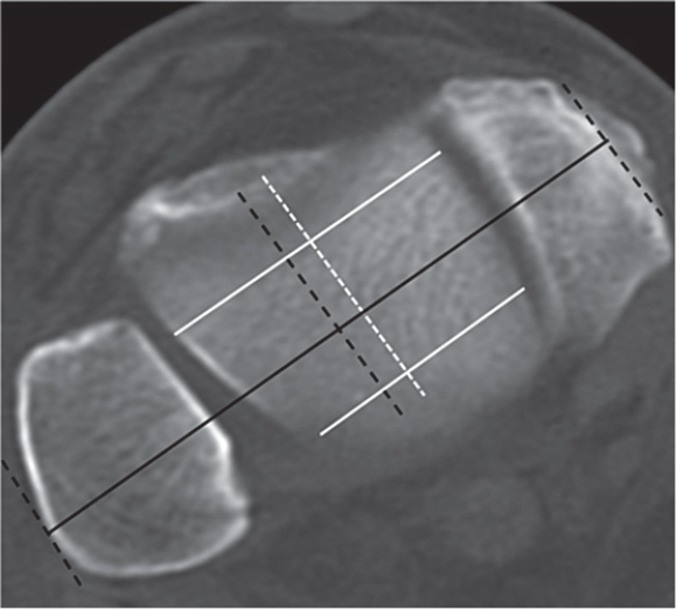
Axial CT scan depicting the intermalleolar distance (continuous black line) and its anterior projection (dotted black line). The white dotted line represents the center of the talar dome originating from the connection of 2 pints located in the middle of its anterior and posterior region (white continuous lines). Note that the center of the intermalleolar distance (dotted black line) is translated laterally with respect to the center of the talar dome.
